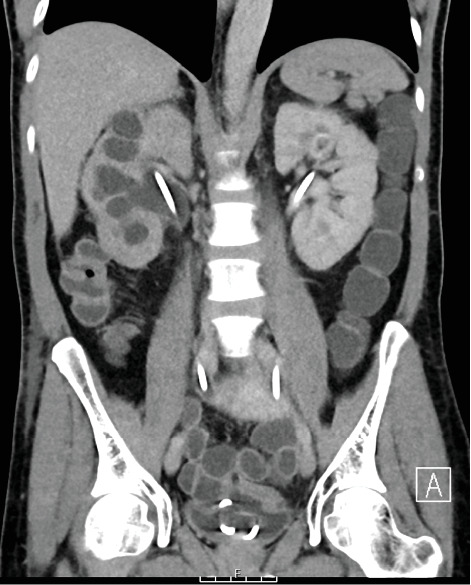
Initial CT urogram (coronal plane) which demonstrates the bilateral stents in situ. A portion of the small bowel where the contrast has extravasated is seen. There is also right pelvicalyceal dilatation.
